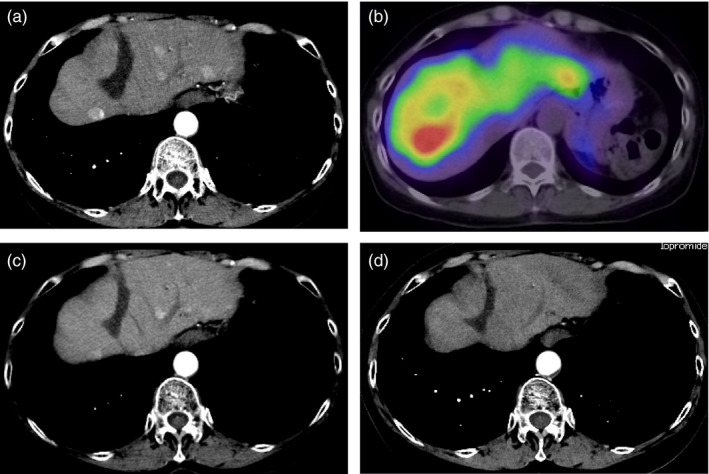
Representative case treated with PRRT. Pancreatic NET with multiple liver metastasis post distal pancreatectomy and multiple liver resection state (WHO 2017 grade 2). (A) Enhanced CT findings revealed multiple hyper vascular lesions in liver before the PRRT. (B) SPECT‐CT imaging before the PRRT. Moderate to intensity uptake revealed multiple liver metastatic lesions. (C) Enhanced CT findings revealed multiple hyper vascular lesions in liver were slightly decrease in size ten weeks after the final PRRT. (D) Enhanced CT findings revealed multiple hyper vascular lesions in liver almost disappeared 28 mo after the final PRRT
